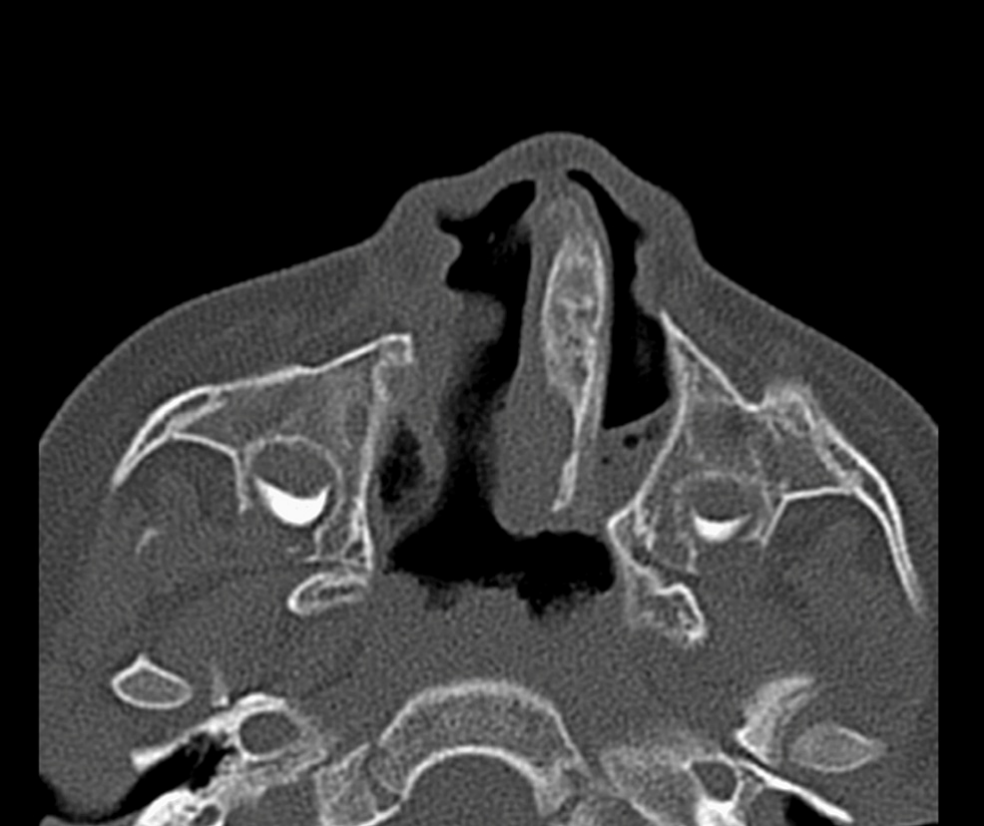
Unilateral choanal atresia This is an axial cut CT scan of a four-year-old male child showing left-sided mixed choanal atresia with a deviated nasal septum to the left side
